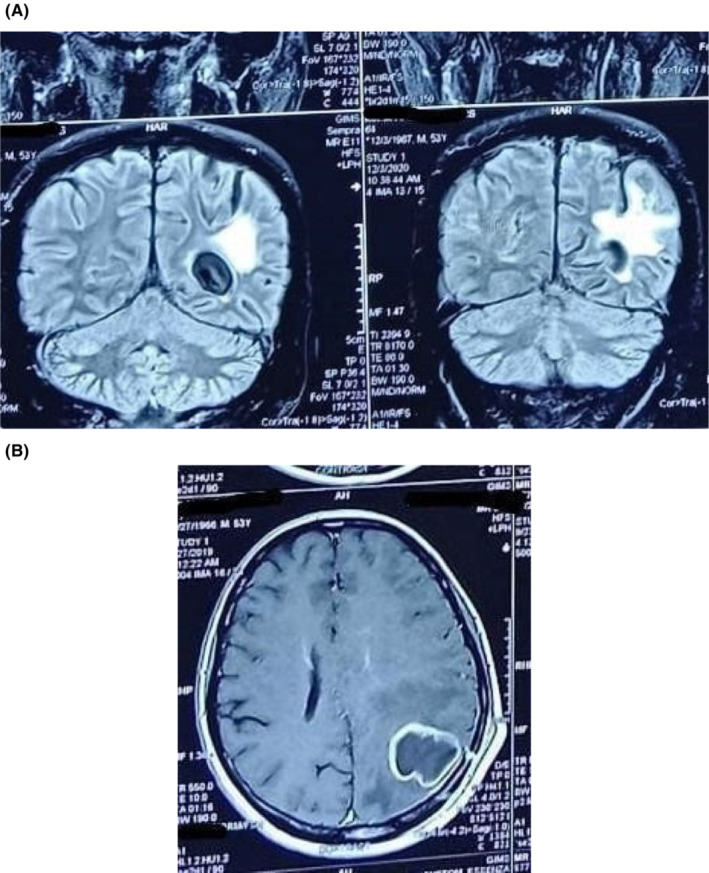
(A and B): MRI showing ring‐enhancing lesion with peripheral edema in the left occipital region
